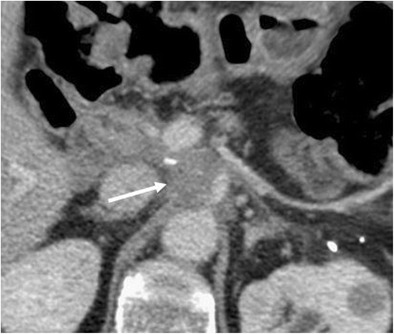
Tumour recurrence. Axial CT image shows the presence of hypodense soft tissue (white arrow) consistent with tumour recurrence, encasing the origin of the caeliac trunk and portal vein
